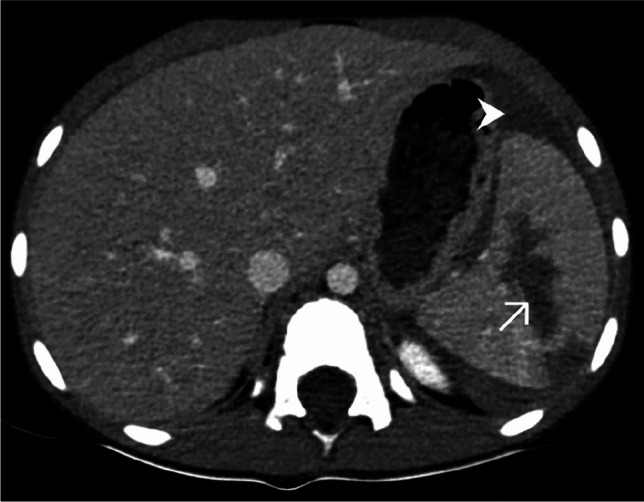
A 5-year-old boy with a history of a ski fall. Axial computed tomography, post-contrast, following a split-bolus protocol, upper-abdominal image (soft tissue window). American Association for the Surgery of Trauma (AAST) grade III splenic laceration seen as a hypodense irregular stripe (*arrow*) and peri-splenic fluid (*arrowhead*)
